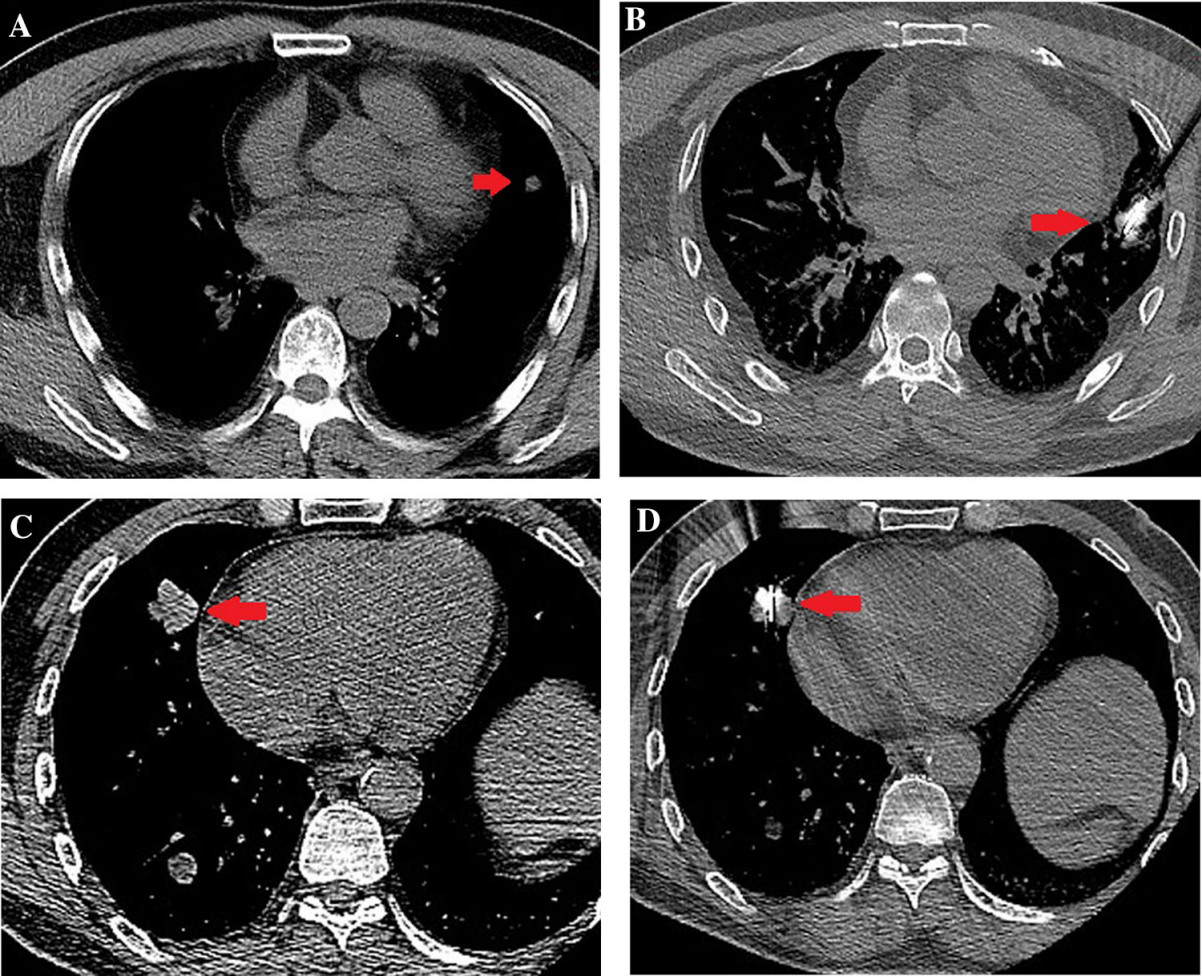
A Chest computed tomography (CT) obtained prior to microwave ablation (MWA) shows a 1.2-cm well-defined round tumor (red arrow) in the left lobe (lesions that were not contiguous to the pericardium). B In the supine position, MWA was performed on the lung tumor adjacent to the pericardium (red arrow). C CT obtained prior to MWA shows a 2.8-cm well-defined round tumor (red arrow) in the right lobe (lesions that were contiguous to the pericardium). D In the supine position, MWA was performed on the lung tumor adjacent to the pericardium (red arrow)
